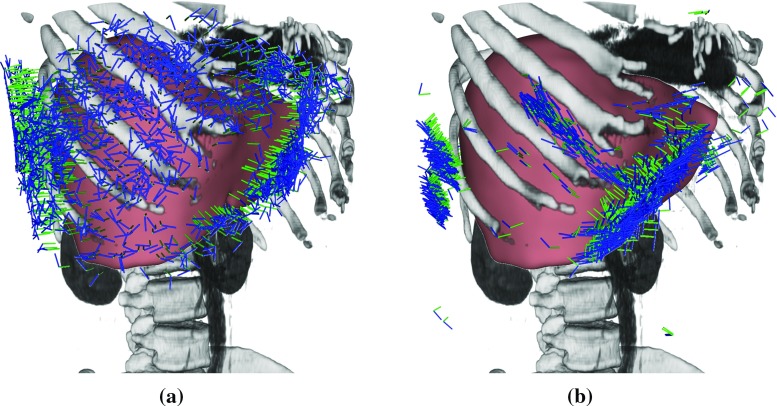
Comparison of single-view poses for the $$N_s$$ target points for one subject from the SLIVER07 dataset [5] together with a render of the underlying CT volume. The orientations of the image axes are visualized with *colored lines*, *green* for axial and *blue* for lateral directions. a shows the plans created naively. The results of our method are shown in (b)
